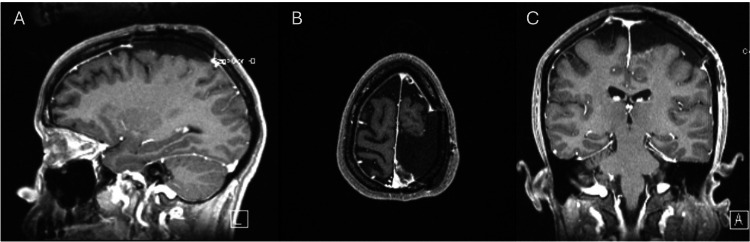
Postoperative MRI. (A) Sagittal MRI shows a gross total resection of the meningioma with a patent superior longitudinal sinus, (B) axial MRI does not show contrast-enhancing of residual tumor, and (C) coronal MRI shows a complete meningioma resection and the absence of cortical edema, which was observed prior to surgery.
